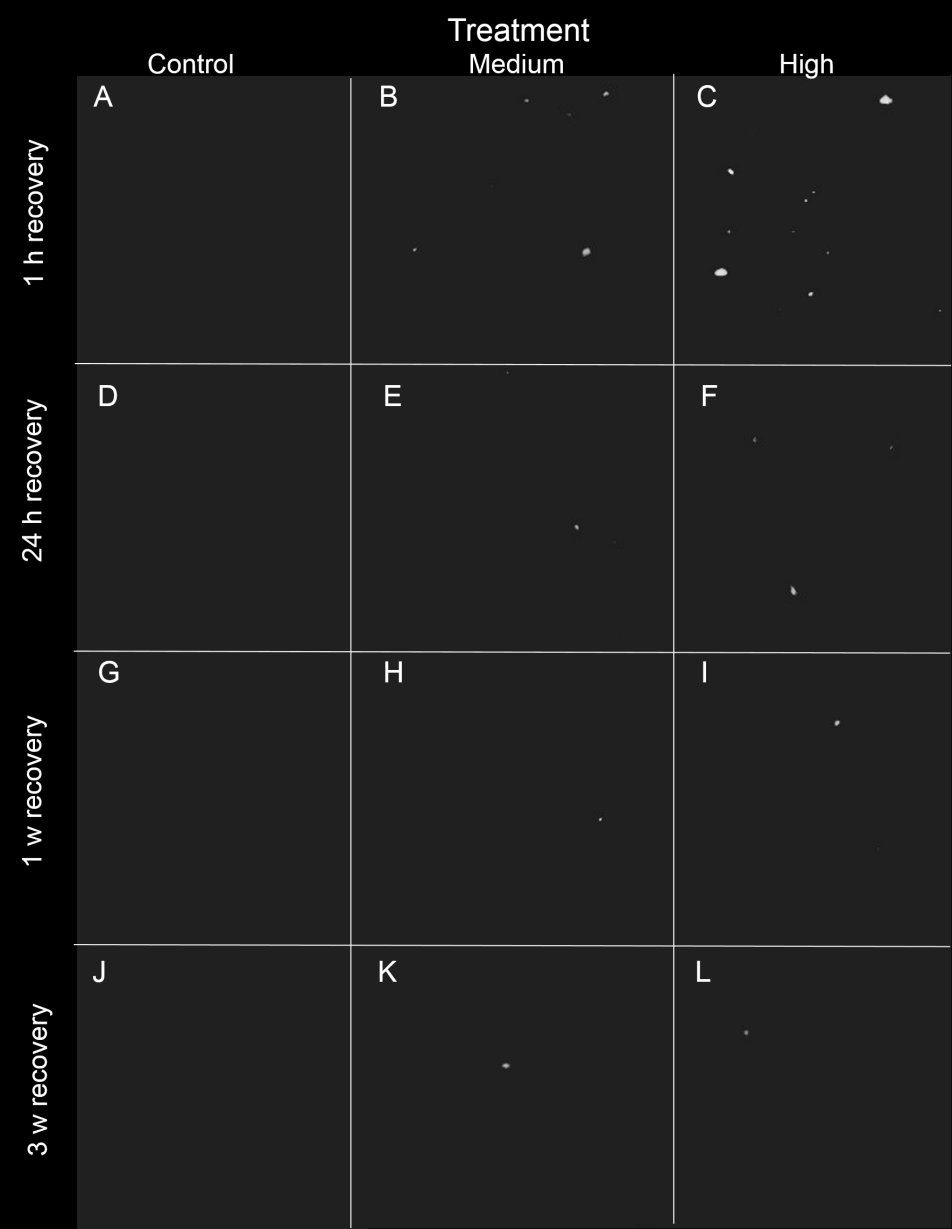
Representative 3D X-ray images of siliciclastic sediments within *I. basta* during the recovery period after 1 h, 24 h, 1 w, and 3 w for the control (0 mg L^−1^), medium (42 ± 8 mg L^−1^), and high (135 ± 30 mg L^−1^) sediment treatments. Sponge tissue (removed via thresholding) is not shown in order to emphasise sediment particles and distribution. As recovery time increases, the sediment (white) becomes less concentrated, and no sediment was observed in the control sponges (no scale is shown because the particles are distributed in 3D).
